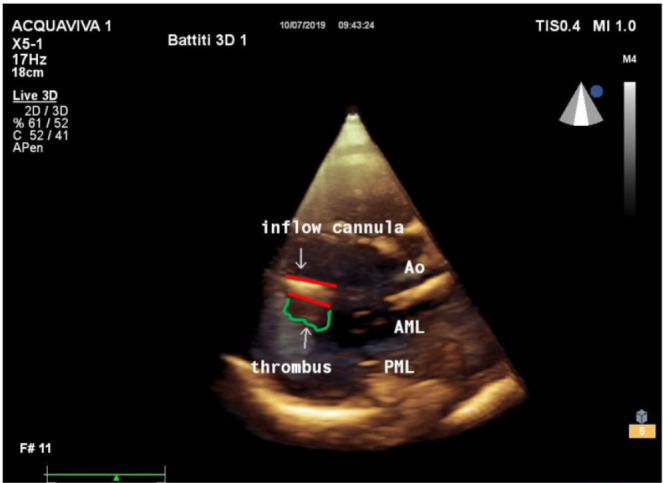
Three-dimensional transthoracic echocardiography. Apical view of the left ventricle: red lines mark both sides of the inflow cannula. The thrombus attached at the bottom side of the cannula is marked in green: the thrombus is not wedging into the cannula, excluding a pre-pump thrombosis. AML=anterior mitral leaflet; Ao=aortic valve; PML=posterior mitral leaflet
